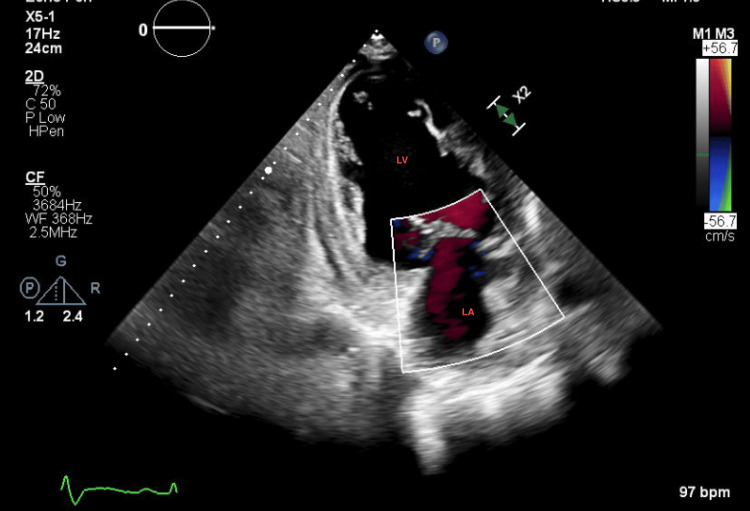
2D transthoracic echocardiogram (two-chamber view) of the patient in March 2021 that did not report trabeculations of the left ventricle
